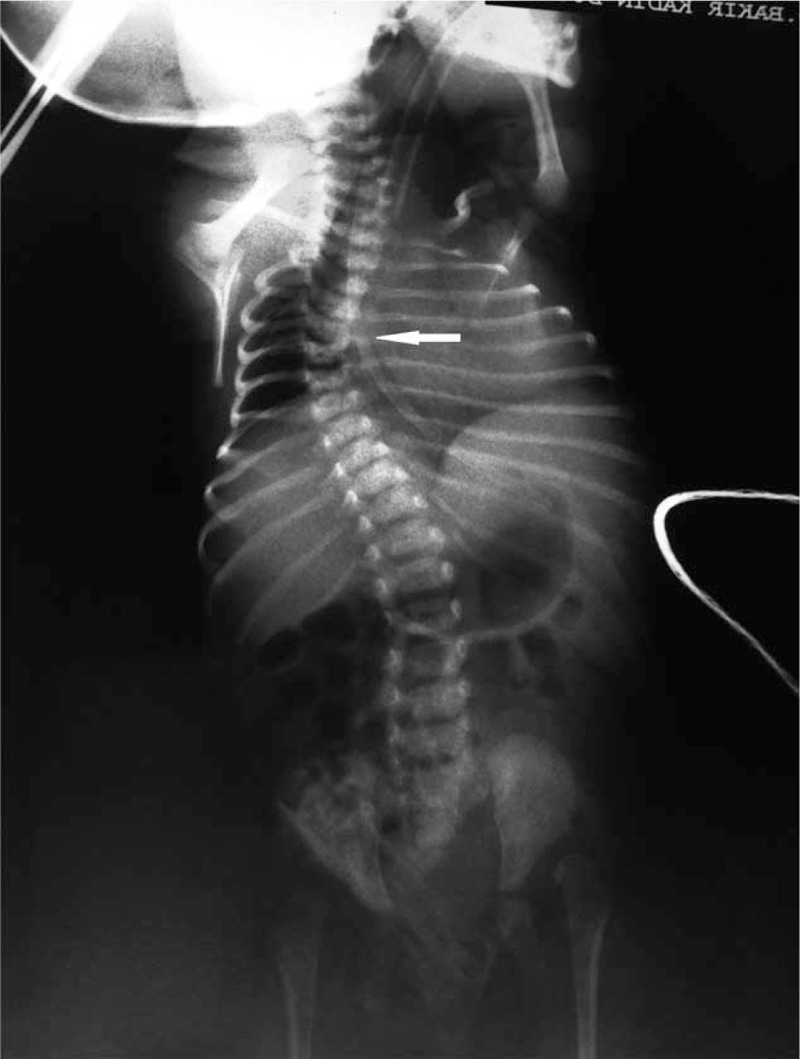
Radiograph of case 10 demonstrates scoliosis in the thoracic spine and reduced lung volume. Note that there is unilateral unsegmented bar (arrow), and vertebrae at the curve region are smaller. Bilateral dislocation of hip is also seen.
